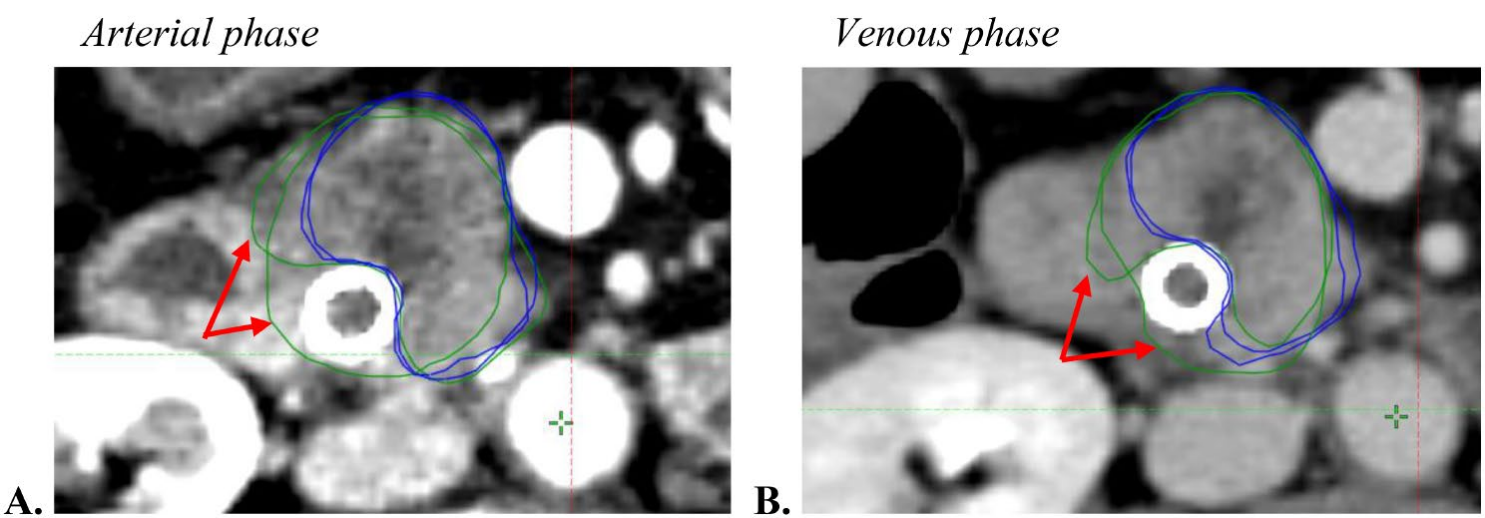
Representative axial CT scans showing intra-observer variability during delineation of the GTV in patient 8. Radiation oncologists’ GTVs are shown in blue and green at the arterial (A) and the venous phases (B). For patient 8 with an intermediate JCI, there was a variability in the delineation of the biliary stent (see red arrow) for the operator in green
